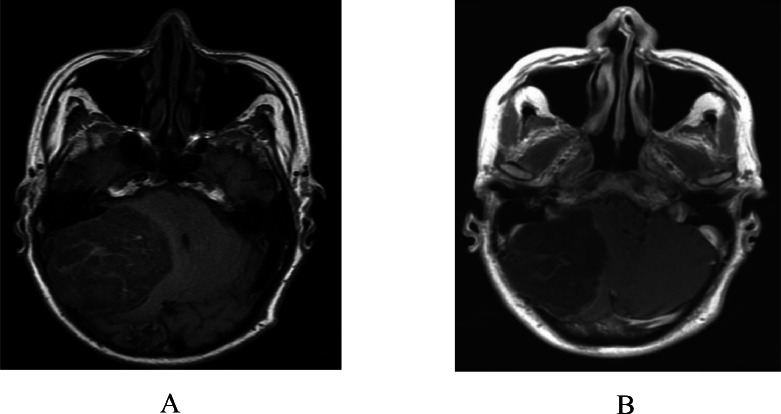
(A) Axial T1 MRI shows the isointense lesion, which, on contrast administration (B), shows subtle contrast enhancement.
